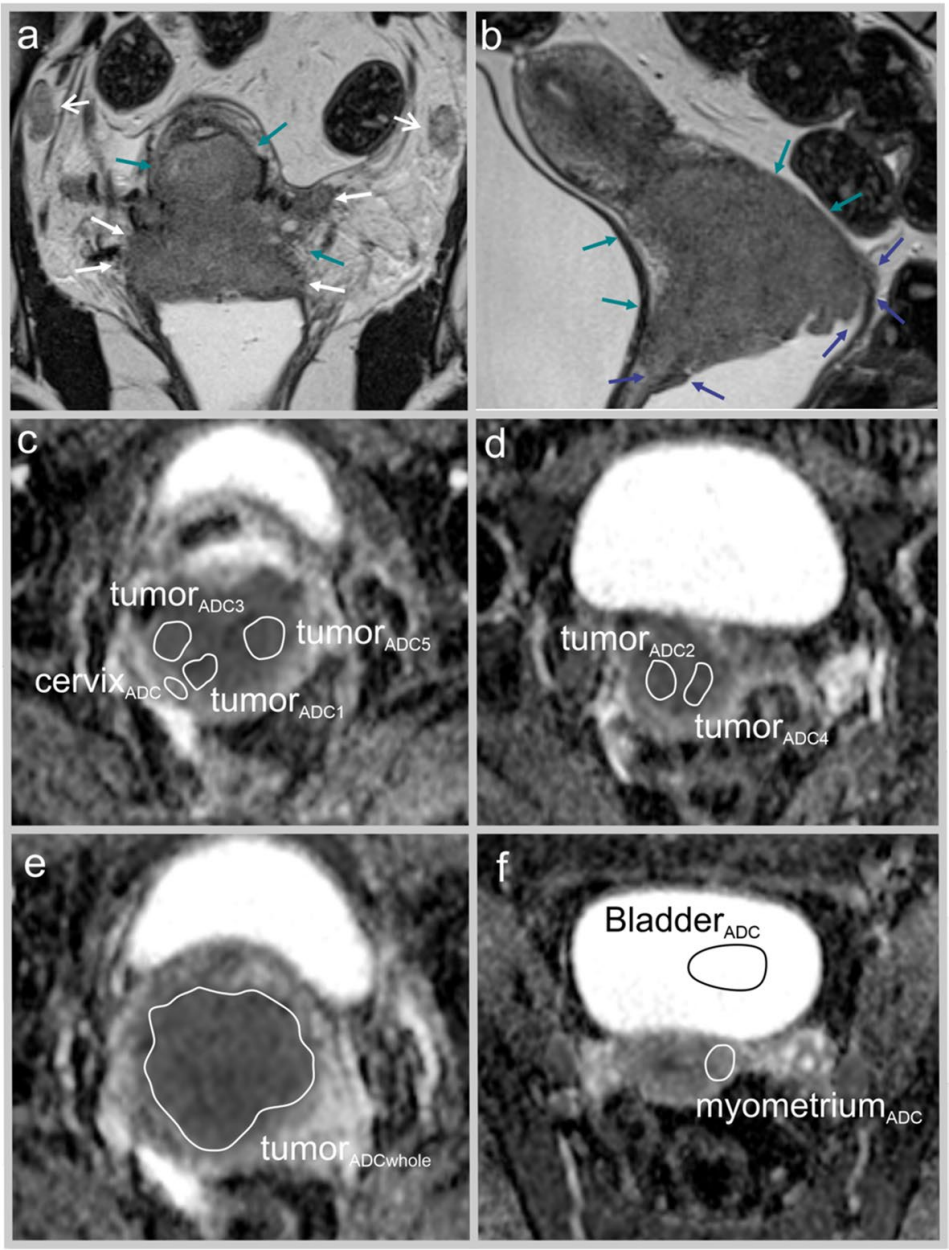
MRI in a 30-year-old woman presenting with a large cervical tumor (squamous cell carcinoma, FIGO (2018) stage IIIC1) prior to treatment with concomitant radio- and chemotherapy. This patient had low myometrium_ADC_/tumor_ADCmean_-ratio at primary diagnosis and she had no signs of recurrence after 8.5 years. Coronal (a) and sagittal (b) T2-weighted MRI depicts a large cervical tumor (green arrows; with maximum diameter 8.5 cm) and disrupted stromal ring (white arrows) (a,b), tumor growth into the upper 2/3 of the vagina (blue arrows) (b) and enlarged (short axis diameter > 1 cm) iliac lymph nodes (open white arrows) (a). Axial oblique (relative to the long axis of the cervix) ADC-maps (c–f) depict restricted diffusion in the primary tumor. The following regions of interest were drawn on the ADC maps: tumorADC_1–5_, tumor_ADCwhole_ bladder_ADC_, cervix_ADC_ and myometrium_ADC._ Tumor_ADCmean_ was calculated as the mean of tumor_ADC1–5_, derived by two independent readers
